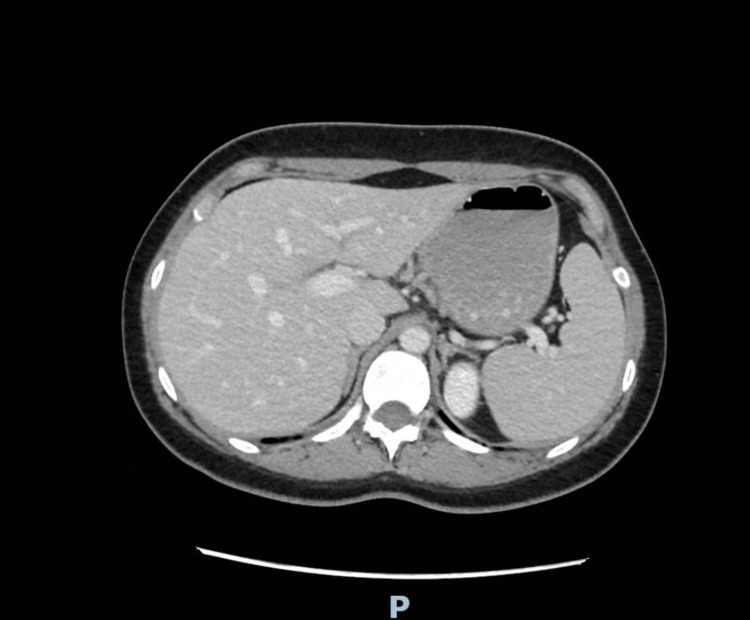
Axial contrast-enhanced CT image of the upper abdomen. No acute hepatobiliary, pancreatic, or upper abdominal abnormality is identified.
